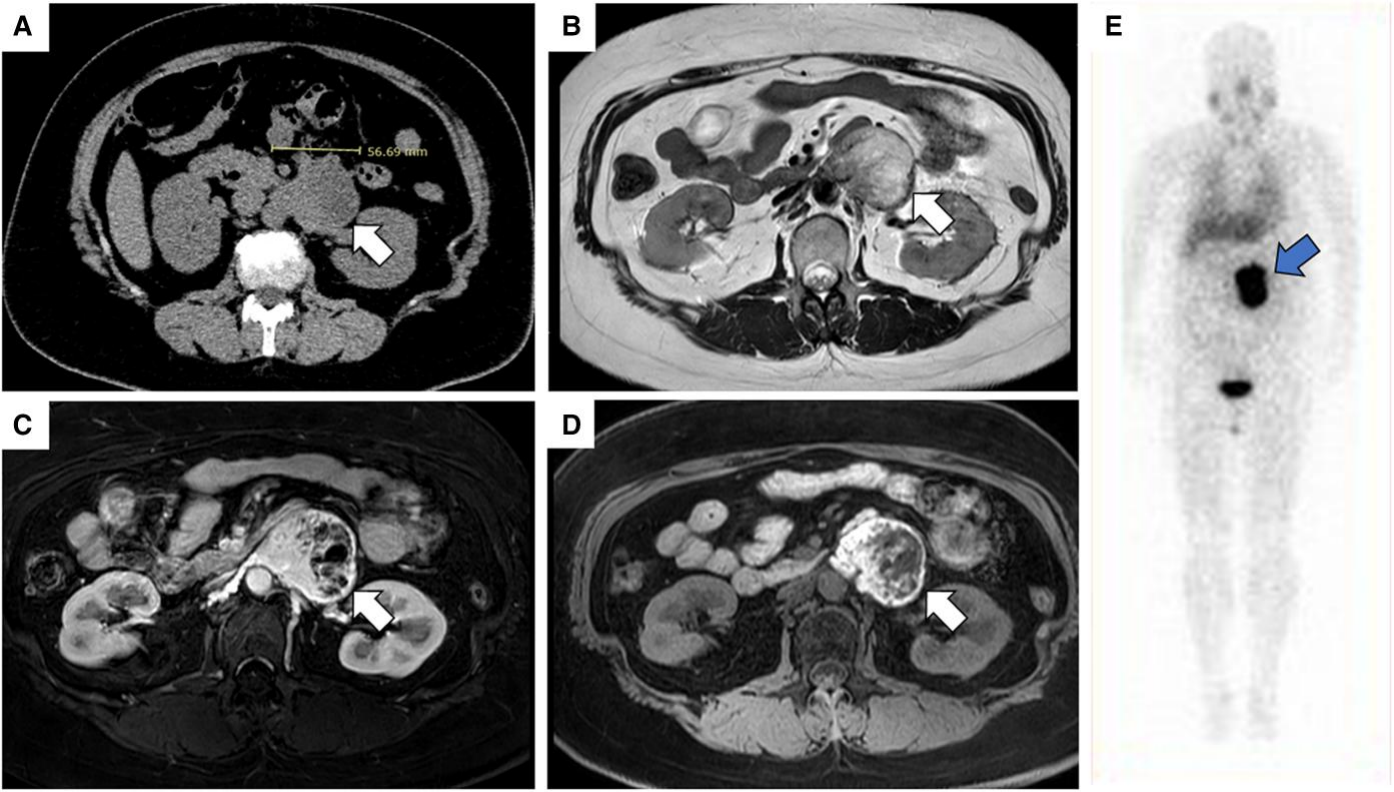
Images displaying a retroperitoneal tumor. A, Plain computed tomography revealed a 56-mm tumor. B, T2-weighted magnetic resonance imaging (MRI) showed a tumor with high intensity. C, Contrast-enhanced MRI showed contrast enhancement in the early phase. D, Fat-suppressed T1-weighted MRI showed a diffuse high intensity. E, Iodine-123 metaiodobenzylguanidine (¹²³I-MIBG) scintigraphy demonstrated an intense uptake consistent with the tumor.
